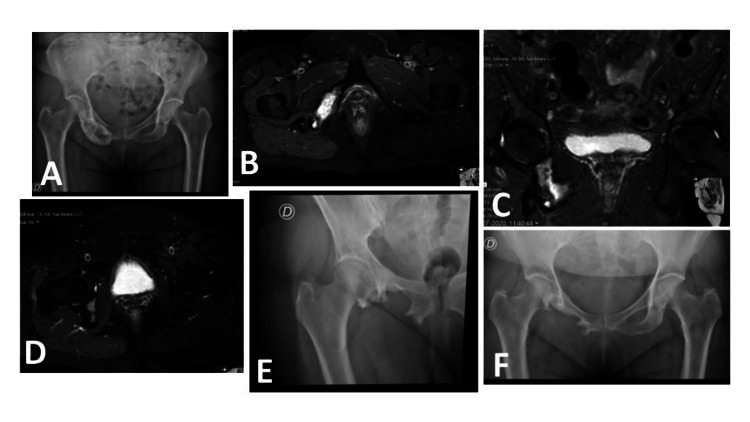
Radiological study of the second patient (A) preoperative pelvic AP view radiograph after treatment with denosumab; (B) preoperative T2 weighted MRI axial cut at the tumor level; (C) coronal; (D) axial cuts of the preoperative T2 weighted MRI showing the relationship of the lesion with the hip; (E) hip AP view; (F) pelvic AP view postoperative radiographs showing the ischiopubic ramus resection. AP: anteroposterior
